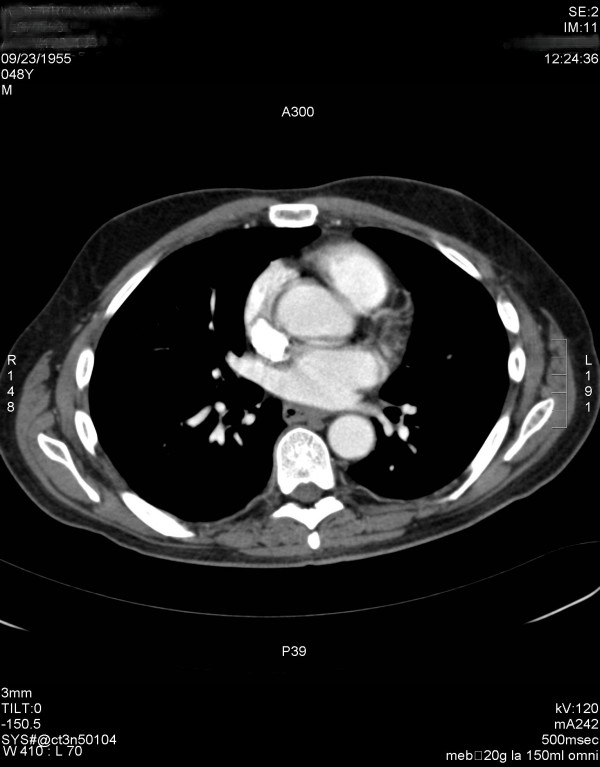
CT Angiogram obtained prior to infusion of rhAPC demonstrating no evidence of pulmonary embolism.
